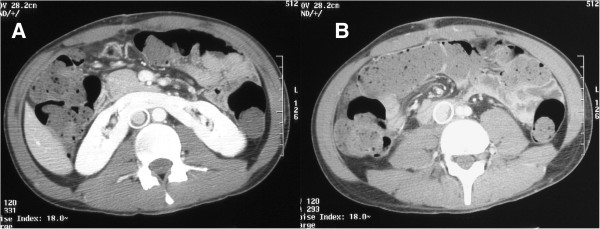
Follow up imaging. The control CT at 1 year (A and B) demonstrates the absence of the lesion and the patency of the prosthesis.
